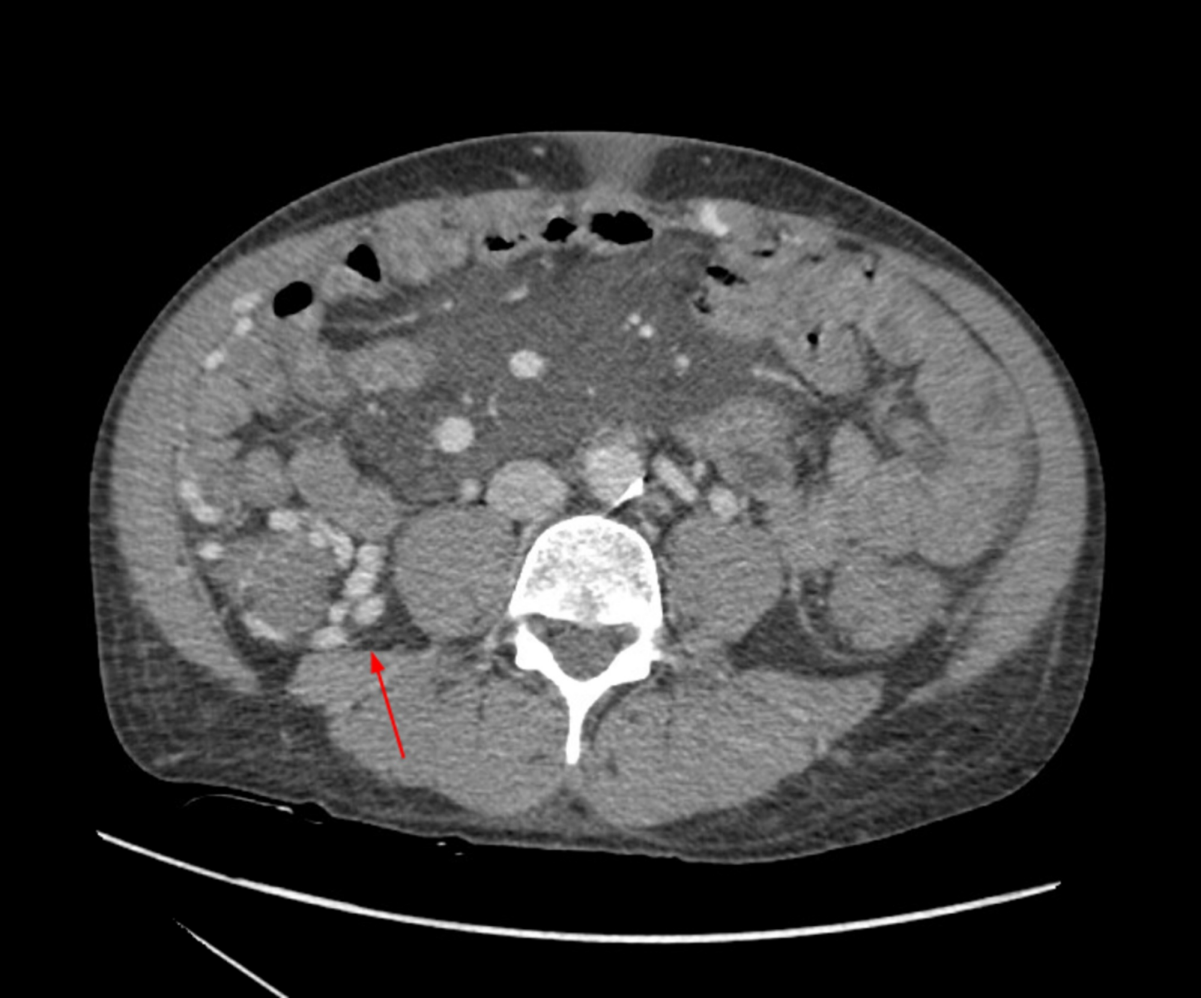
Arterial phase CT angiography demonstrating right cecal varices (red arrow) without evidence of active hemorrhage. Contrast evident within venous system during arterial phase.
